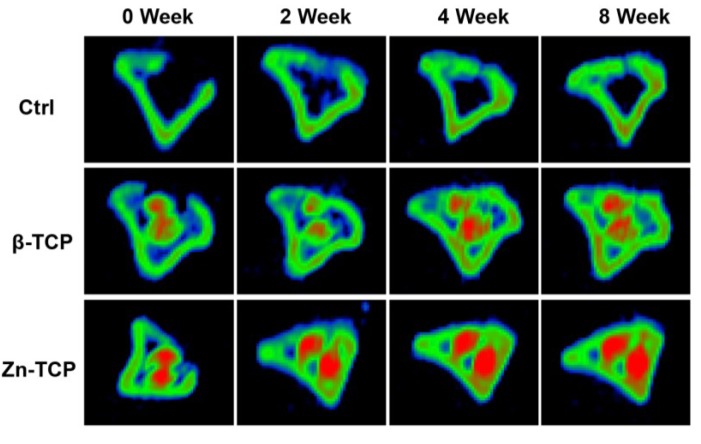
CT images corresponding to the empty control (top), β-TCP (middle) and Zn-TCP (bottom) showing the progressive restoration of cortical and trabecular bone over eight weeks at the defect. Zn-TCP showed complete cortical bone restoration at four weeks and continuous growth of trabecular bone. The sample materials are highlighted in red.
